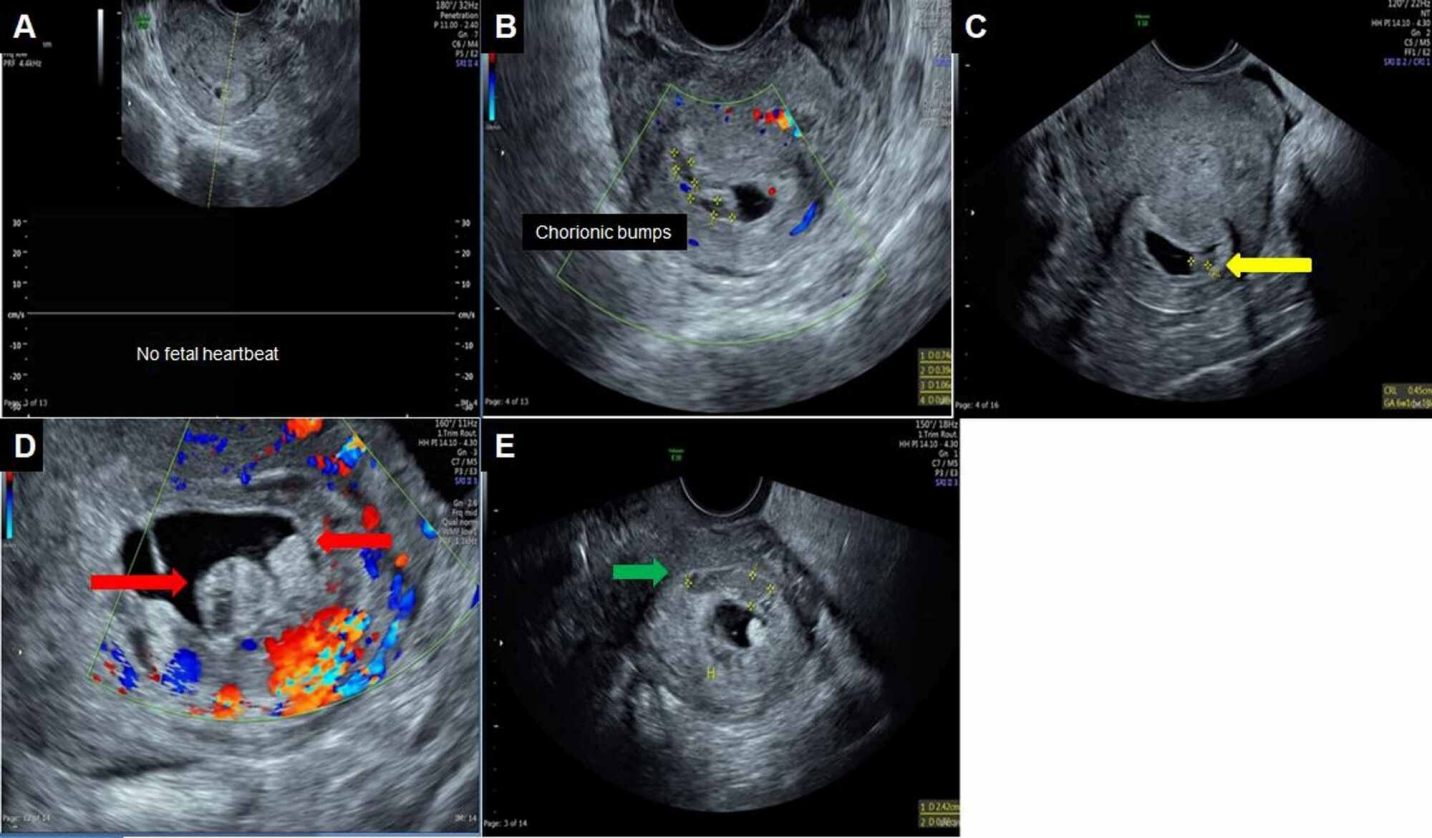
Transvaginal ultrasound (A) The first ultrasound was done at presentation, and it shows a 3 mm gestational sac with no fetal heartbeats. (B & C) There is a small lesion protruding from the gestational sac wall into the cavity, with central hypoechoic and peripheral hyperechoic rim, and no vascularity is consistent with the chorionic bump. (D) A one-month follow-up scan, showing two protrusions from the chorion into the gestational sac with a central hypoechoic and peripheral hyperechoic areas with no vascularity on color Doppler were suggestive of chorionic bumps (these lesions were initially mistaken as gestational sac). (E) A heterogeneously hypoechoic area (green arrow) outside the chorion with no internal vascularity is a subchorionic hematoma.
